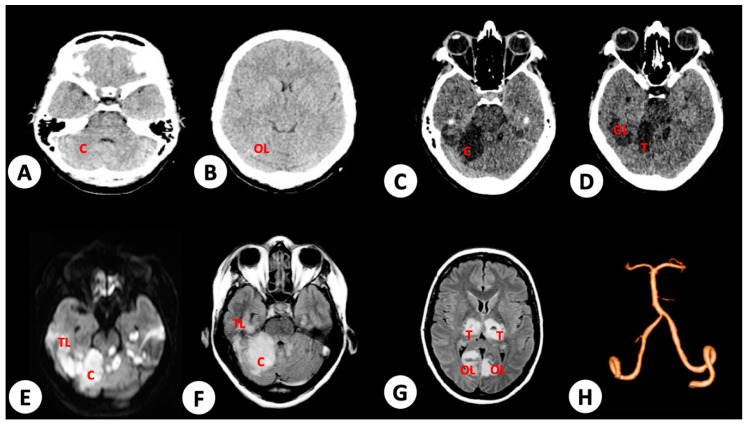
Brain radiologic imaging of patient 1. (A) No evidence of mass or vascular lesions in cerebellum or (B) occipital lobes (OL) in brain computed tomography (CT) immediately after seizure and decerebrate posturing events (10 days post onset of dengue symptoms). (C) T1 weighted image from brain nuclear magnetic resonance (NMR) performed 24 h after occurrence of disorientation and seizures, with evidence of ischemia in cerebellum (C) (11 days post onset of dengue symptoms). (D) Ischemic areas in thalamus (T) and occipital lobe. (E,F) Extensive ischemic infarct in cerebellum and parietal lobe (PL), in diffusion-weighted and fluid-attenuated inversion recovery (FLAIR) image from brain NMR performed 24 h after occurrence of acute clinical events (disorientation and seizures), respectively. (G) Ischemic infarct in thalamus and occipital lobe. (H) No evidence of vessel lesions, including basilar and vertebral arteries responsible to vascularization of injured areas in brain angiographic study performed 24 h after seizures.
